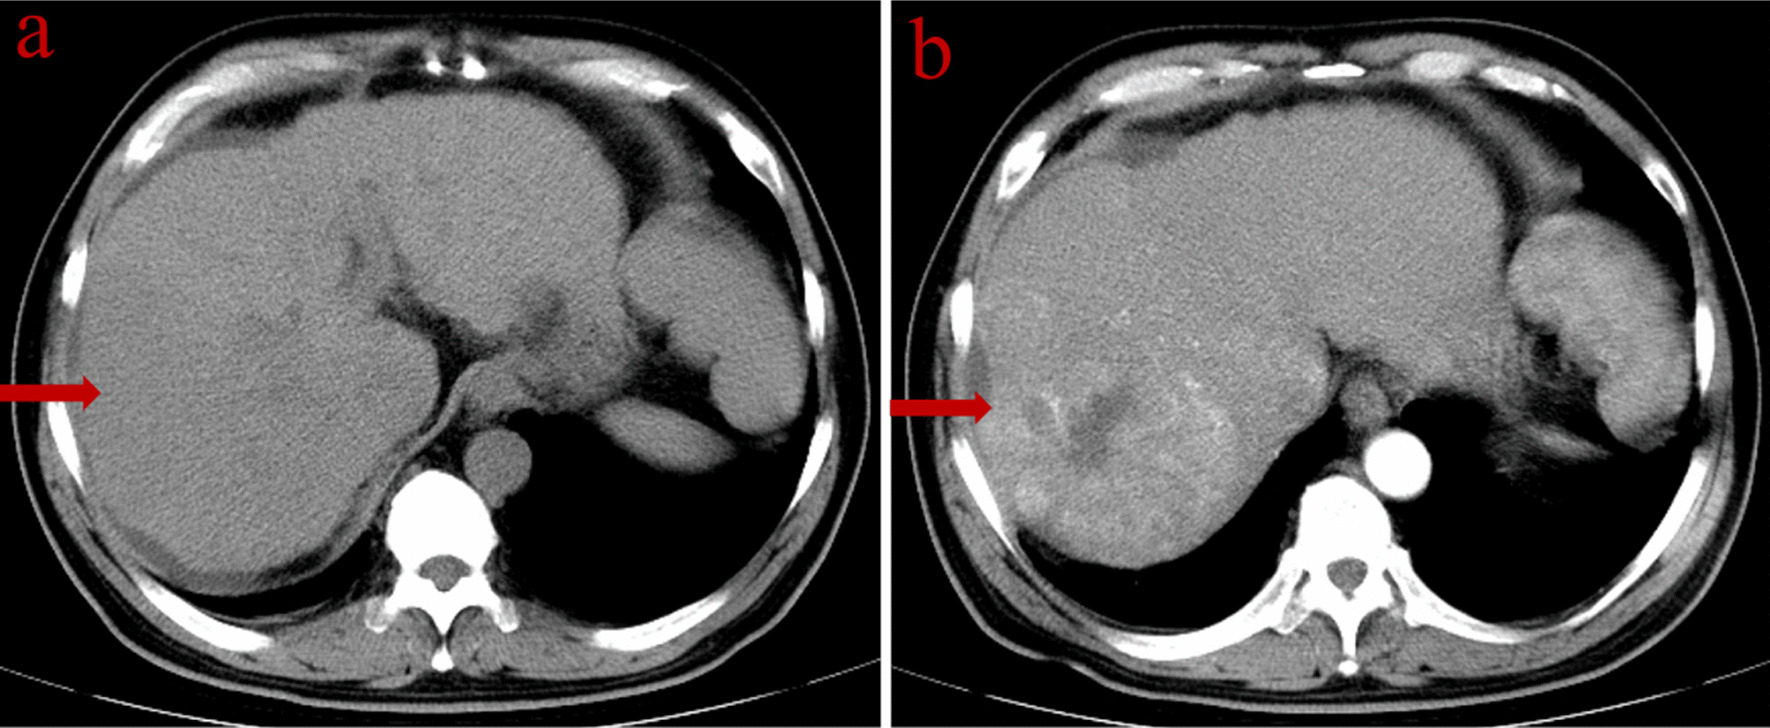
a CT scan showed an irregular low-density soft tissue mass in S6. b In the arterial phase, the mass was enhanced homogeneously, with a size of approximately 6.5 cm × 5.8 cm
